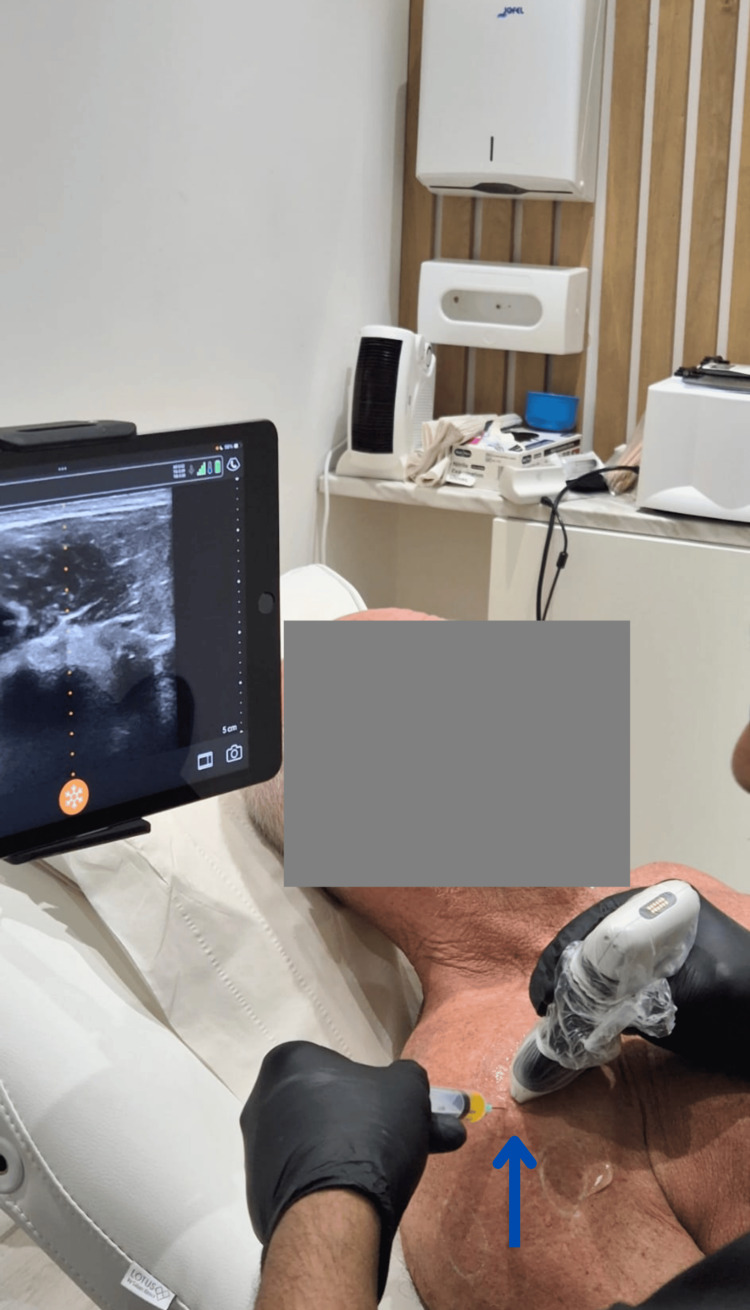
Anterior subacromial approach for injection of yellow PRP under ultrasound guidance (blue arrow) into the proximal long head of the biceps tendon PRP: platelet-rich plasma
